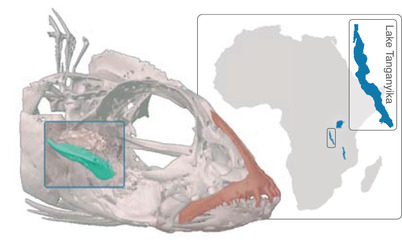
Three‐dimensional reconstruction (based on micro‐computed tomography) of the head of the cichlid species *Neolamprologus furcifer* from Lake Tanganyika (inset). The upper oral jaw (premaxilla) as part of the oral jaw apparatus is highlighted in red. A section of the skull was virtually removed (blue box) to uncover the pharyngeal jaw apparatus, with the lower pharyngeal jaw bone being highlighted in green.
